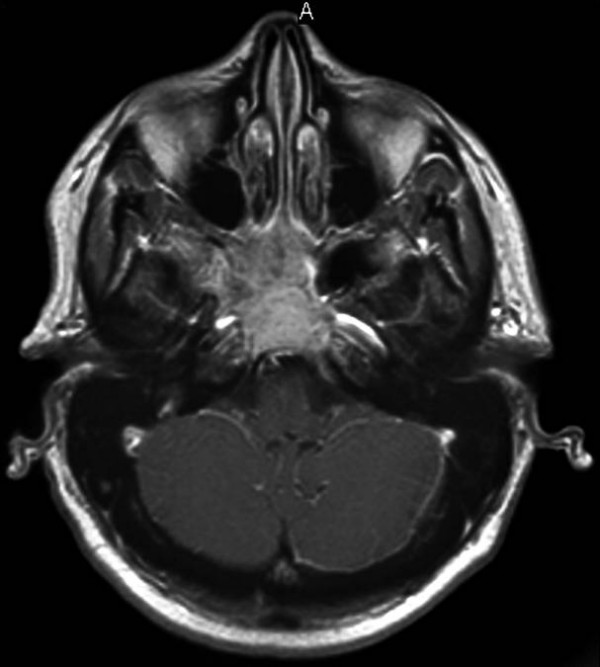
Magnetic Resonance Imaging of the Brain: a large mass in the clivus extending anteriorly to involve the nasopharynx and sphenoid sinus and posteriorly to destroy the clivus and lie anterior to the pons.
